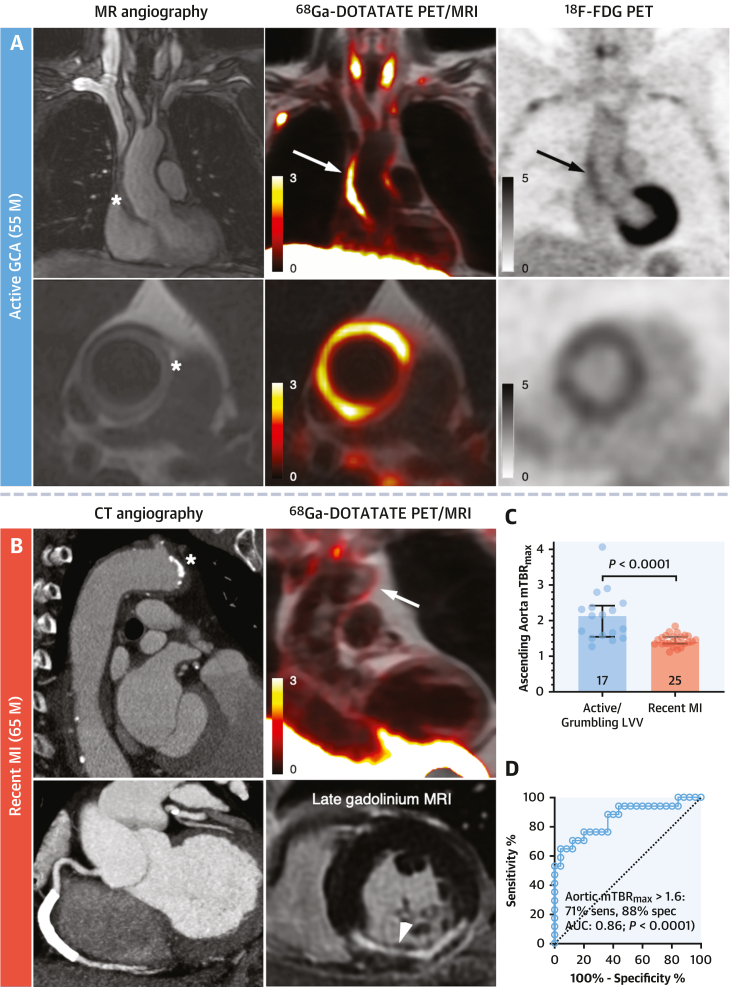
Vasculitis vs Atherosclerotic Inflammation Aortic SST_2_ PET/MRI signals (white arrows) in patients with (A) active GCA (treatment naive at baseline imaging) and aortic thickening (asterisk) and (B) recent MI with aortic atherosclerosis (asterisk) and inferior infarction (arrowhead; note the infarct-related myocardial PET uptake). Contemporaneous ^18^F-FDG PET images in A show aortic uptake (black arrows). (C) Quantitative comparison of aortic mean SST_2_ PET TBR_max_. (D) Receiver-operating characteristic analysis of SST_2_ PET mean TBR_max_ for differentiating active/grumbling from inactive LVV. Image scale bars indicate SUV; error bars in C indicate median (IQR). M = male; other abbreviations as in Figures 1 and 2.
